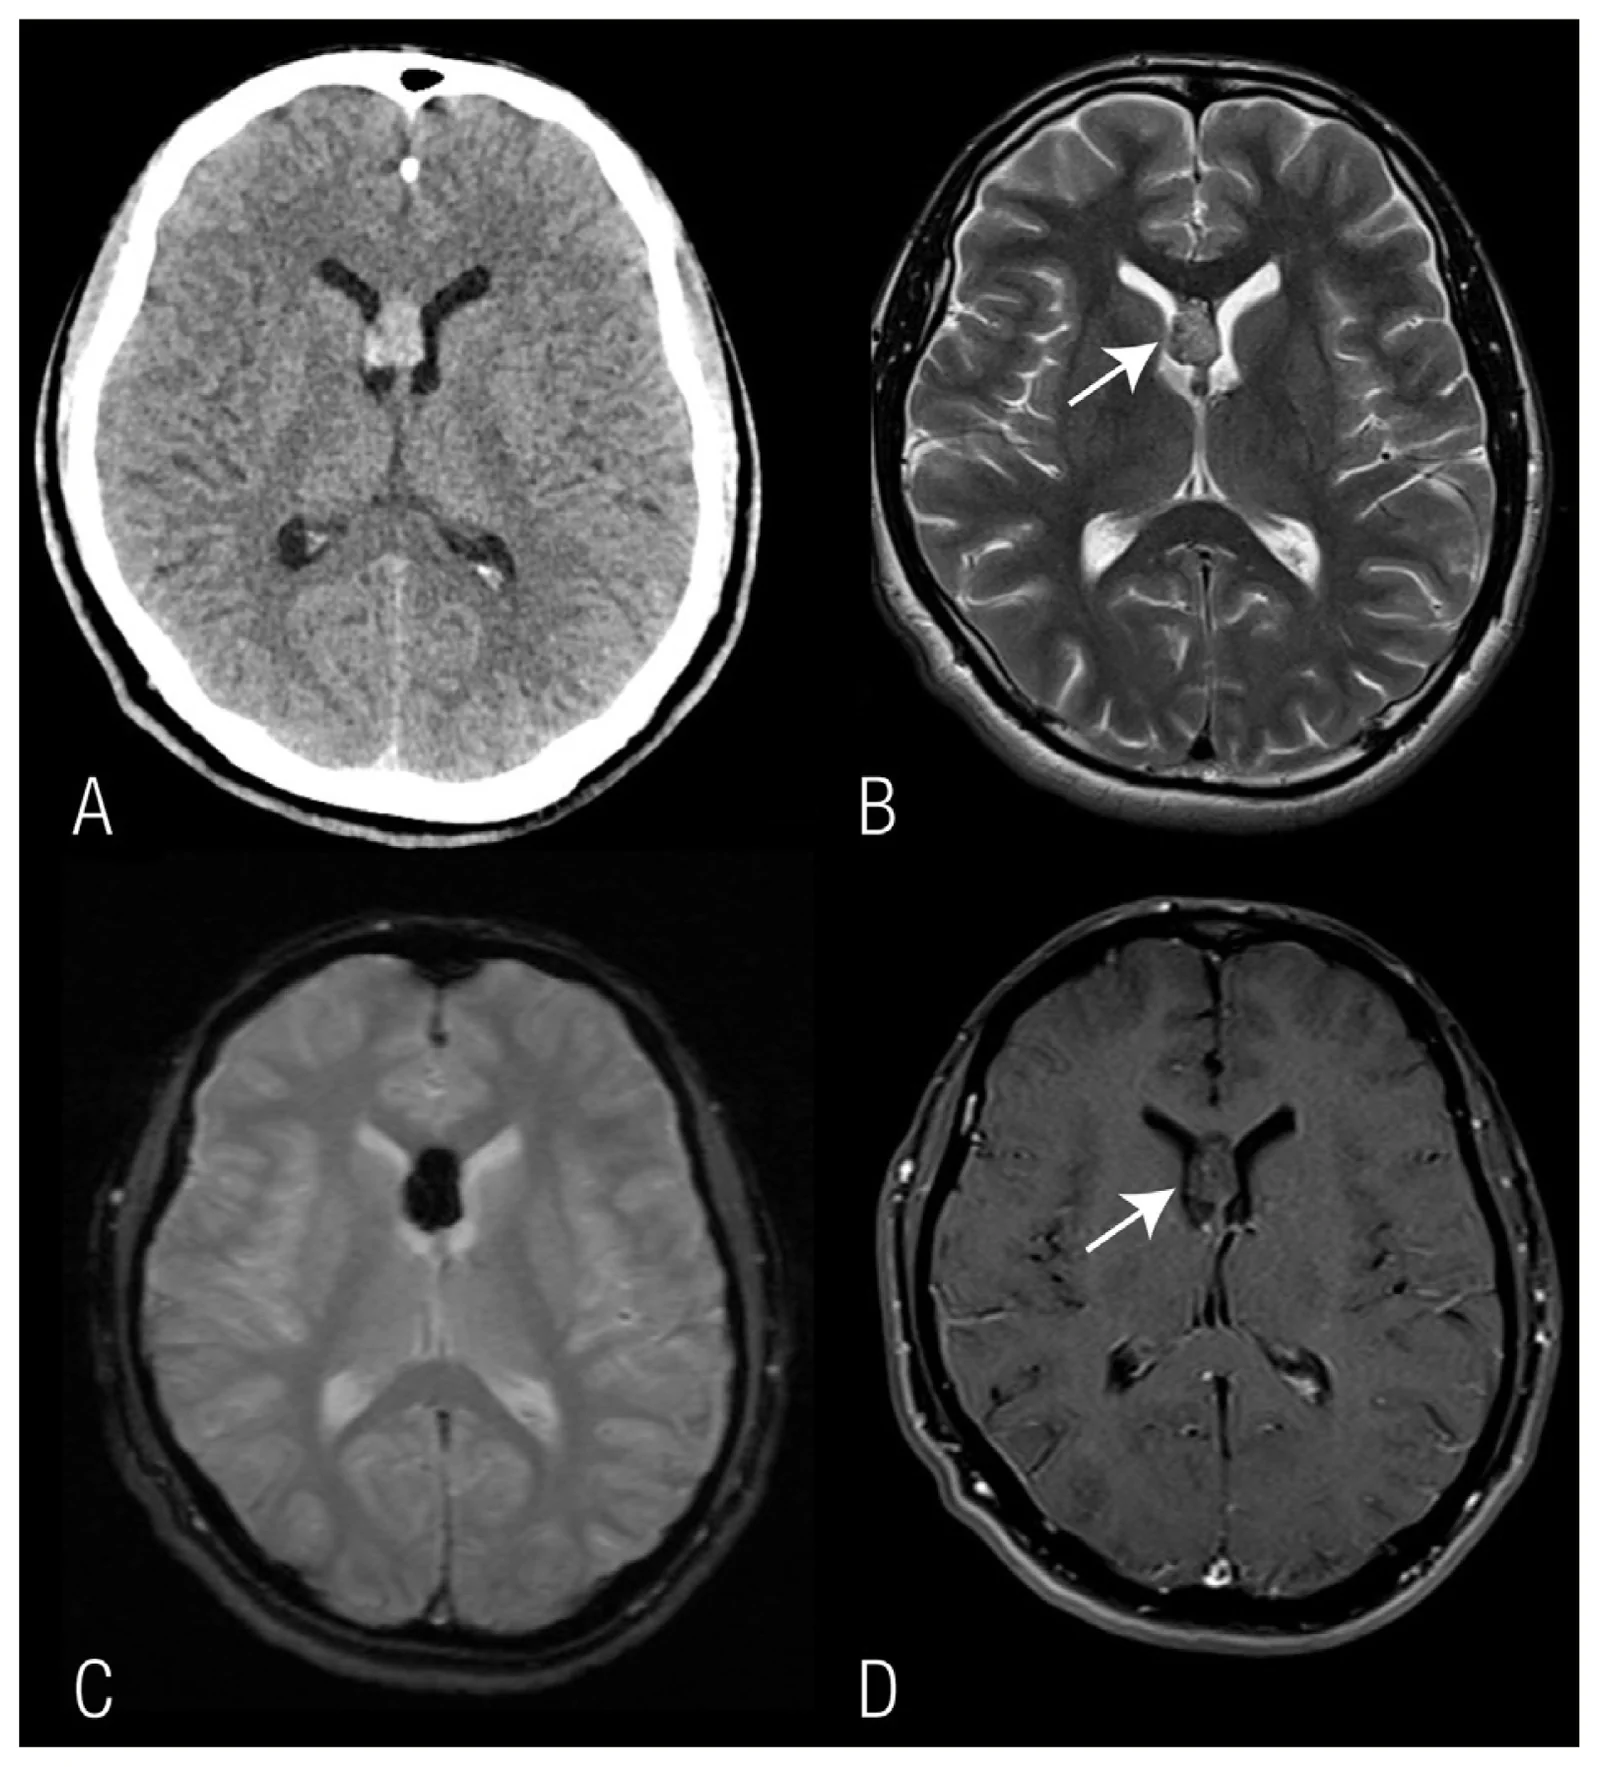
A: Axial non-enhanced computed tomography image showing a hyperdense lesion of the septum pellucidum at the level of the frontal horns, bulging bilaterally into the lateral ventricles. B: Axial T2-weighted image showing the lesion to be T2 hyperintense with a bubbly appearance. C: T2* gradient echo image showing significant blooming at the site of the lesion. D: Post-contrast axial T1-weighted image showing no significant enhancement.
